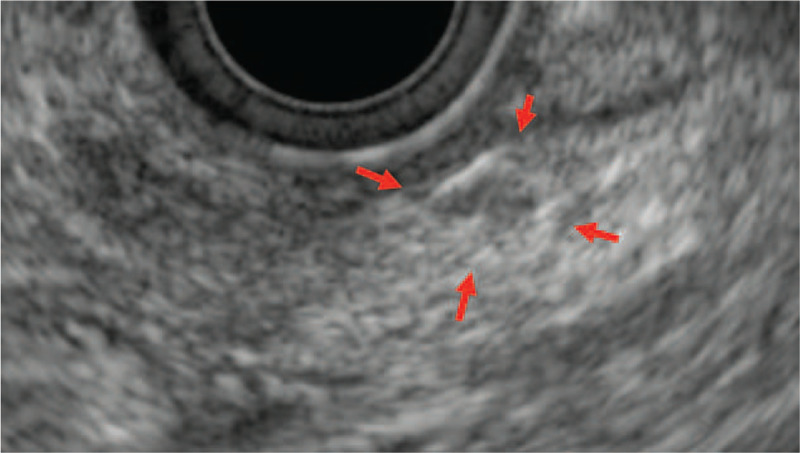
Endoscopic ultrasonography revealed a 6 mm nummular hypoechoic mass, the margin was relatively clear and smooth.
